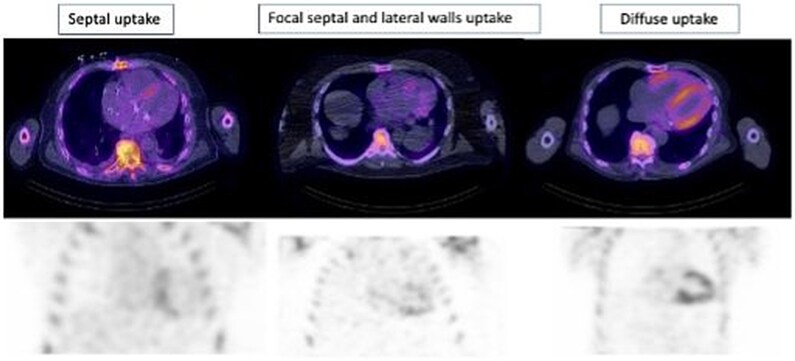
Patterns of uptake with positive conversion. Upper row: (Left panel) Focal uptake in the septum; (Middle Panel) focal uptake in the septum and lateral wall; (Right Panel) diffuse uptake, extending to the right ventricular free wall. Lower row: Corresponding planar data, demonstrating radiotracer uptake equal or greater than rib uptake (grade ≥1), with similar distribution demonstrated on SPECT/CT images.
